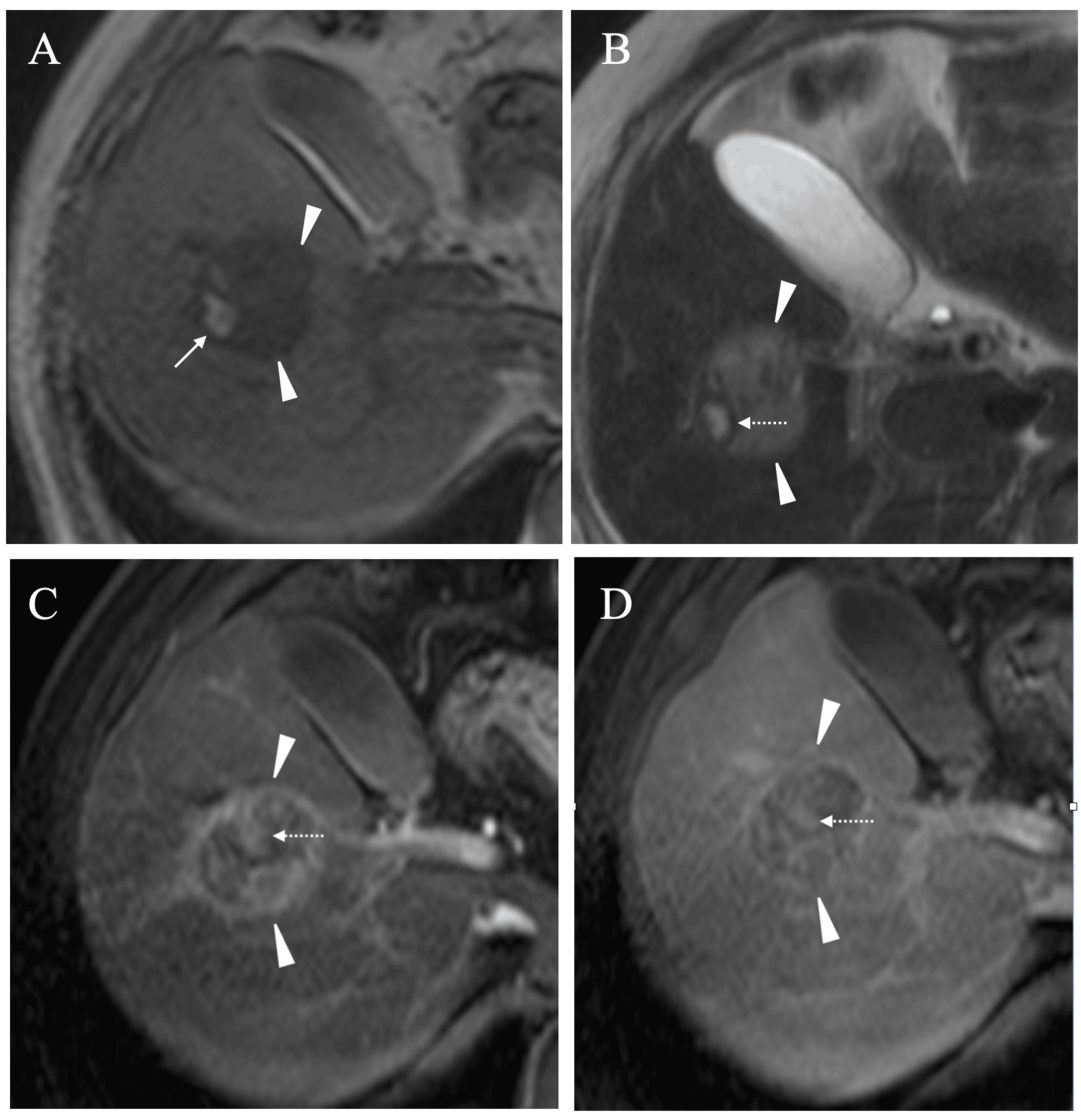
Magnetic resonance image (MRI) findings A. T1-weighted images showed that the mass showed low signal intensity (arrowheads) and had a small high-signal intensity area (arrow) in the mass borders. B. T2-weighted images showed that the mass showed slightly high signal intensity (arrowheads) and had a small high-signal intensity area encircled by a very low signal intensity ring (dashed arrow). C. T1-weighted images 31 seconds after the contrast medium injection showed a weak rim enhancement (arrowheads) and contrast effect directing toward the inside of the tumor (dashed arrow). D. T1-weighted images 120 seconds after the contrast medium injection still showed a weak rim enhancement (arrowheads) and a slight intra-tumoral enhancement (dashed arrow).
